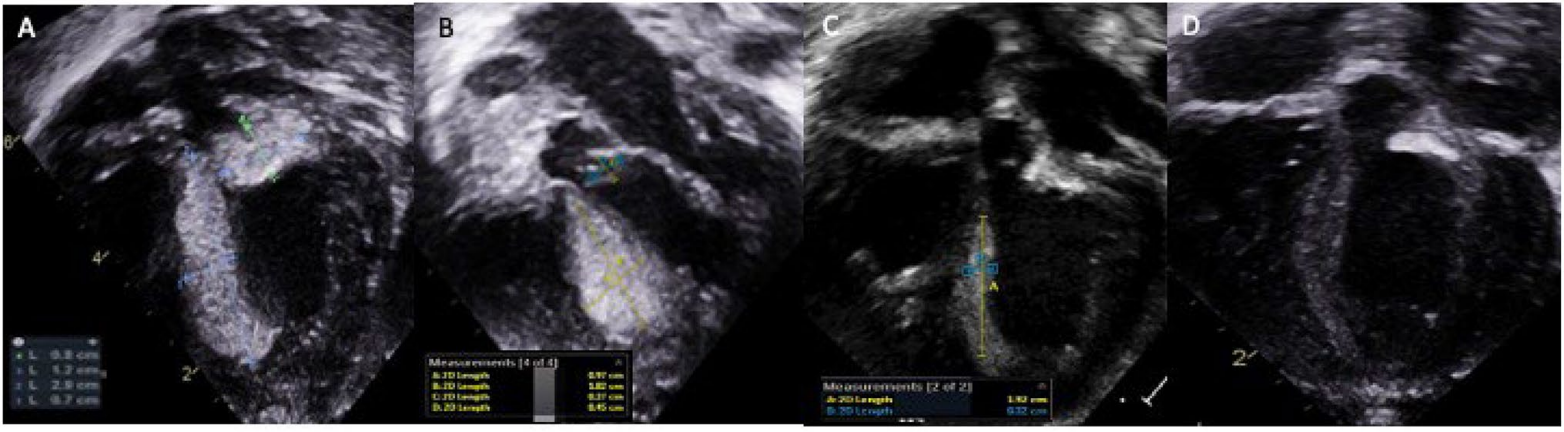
Serial images of Transthoracic Apical five-chamber view in case 2: A at birth; B 8 days post surgical resection of LVOT tumour and Sirolimus therapy; C 2 weeks post Sirolimus; D 4 weeks post Sirolimus. Note: at 2 weeks post mTORi, the tumour at interventricular septum (IVS) has regressed 35–55% compared initial presentation. Same tumour was completely regressed at weeks of Sirolimus therapy
